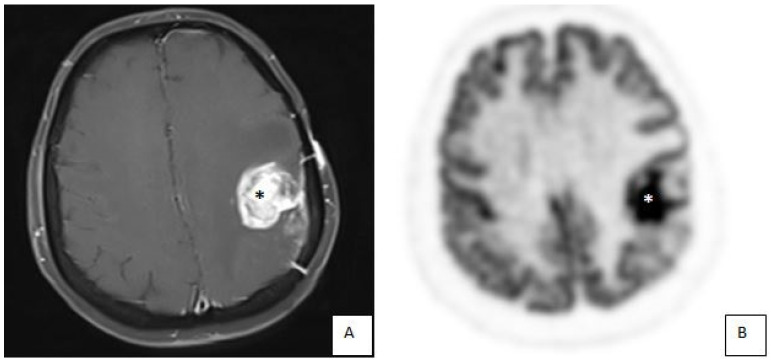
Case 3. Magnetic resonance imaging (MRI) (A) revealed an enhancing mass lesion (black asterisk) in the left frontoparietal lobe. A hybrid PET/MRI image (B) showed a hot spot (white asterisk), suggesting recurrent glioma.
